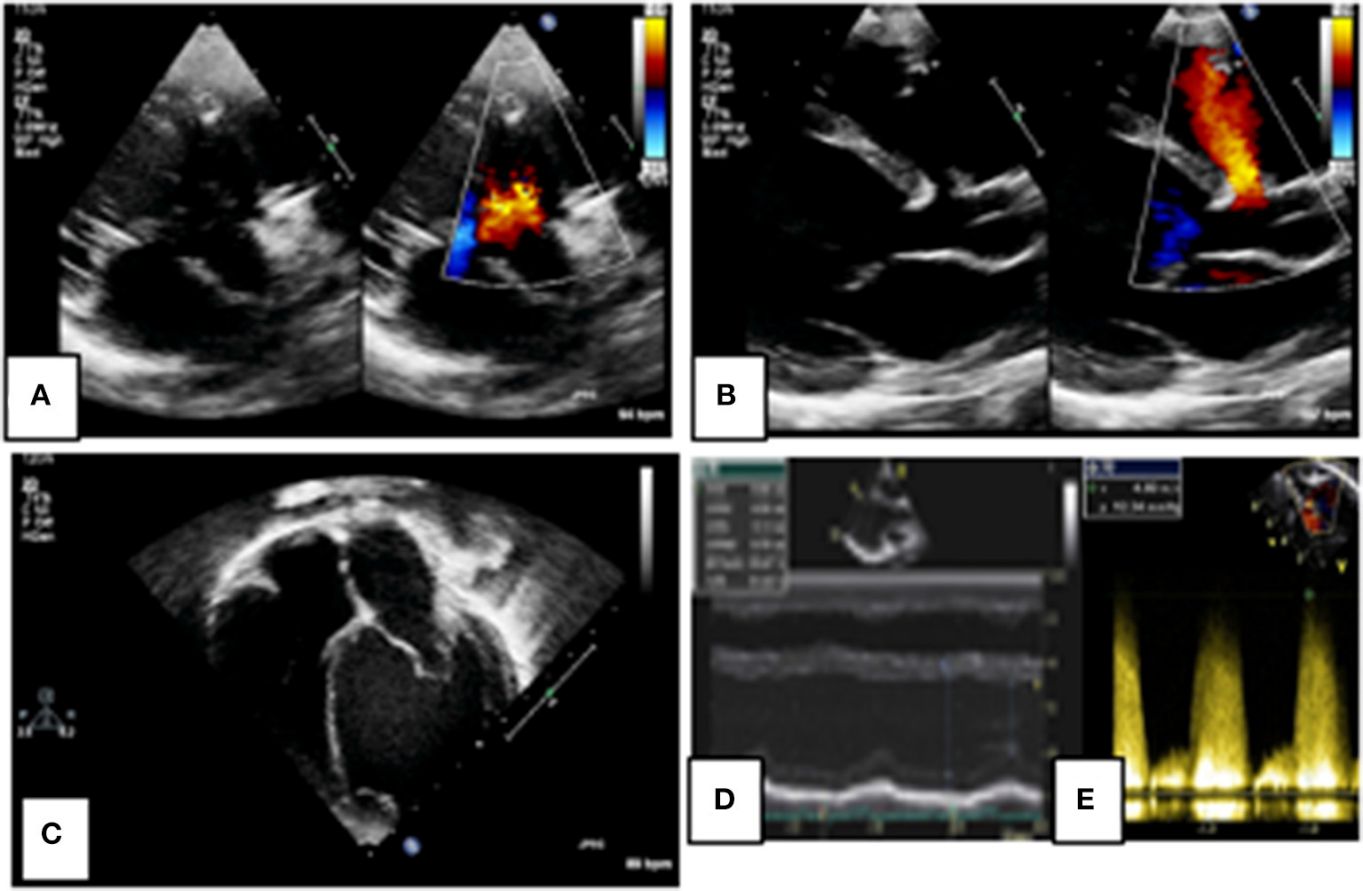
2D echocardiographic assessment of a peri-membranous ventricular septal defect (VSD). Figures (A,B) define the site and size of the lesion as a small peri-membranous VSD with a left-to-right shunt on color Doppler. There is evidence of left atrial and ventricular dilation in figure (C) indicating a significant left-to-right shunt, as measured on M-mode (D). The shunt velocity is high on spectral Doppler indicating favorable hemodynamics for VSD closure (E).
